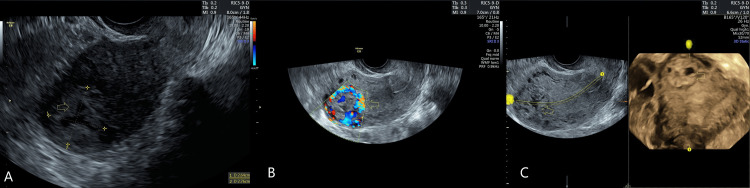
Ultrasound images shows the cornual ectopic. (A) shows the cornual ectopic. (B) shows the increased vascularity of the mass. (C) shows the three-dimensional ultrasound of the mass.
